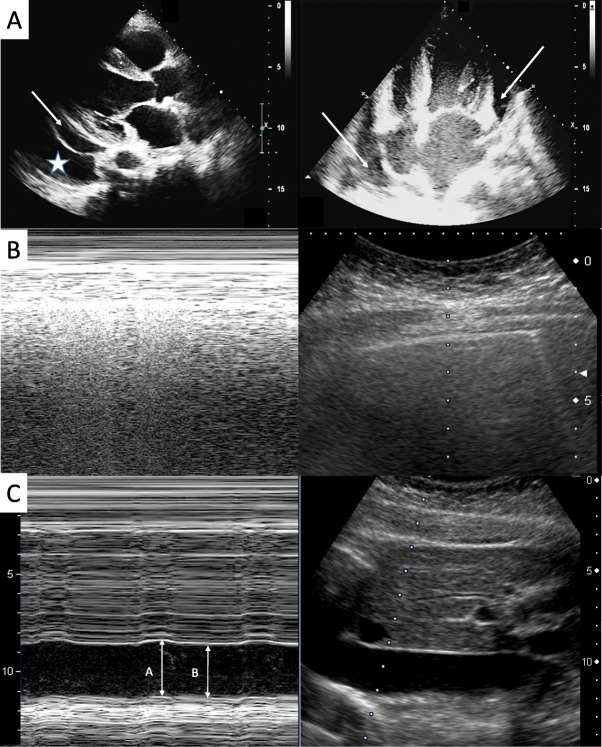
Point-of-care ultrasound. Machine: Toshiba Aplio MX SSA-780A. Probe: micro convex (cardiac ultrasound), convex (lung and IVC ultrasound) a Focus cardiac ultrasound with long-axis view and four chamber view. Large amount of pericardial effusion (arrows). Pleural effusion (star). b Lung ultrasound. Normal lung sliding without prominent B lines. Normal lung pattern on M-mode: the seashore sign. c IVC ultrasound. The IVC diameter was measured as A (3 cm, during expiration) and B (2.7 cm, during inspiration). M-mode showed minimal change during respiratory cycle.
